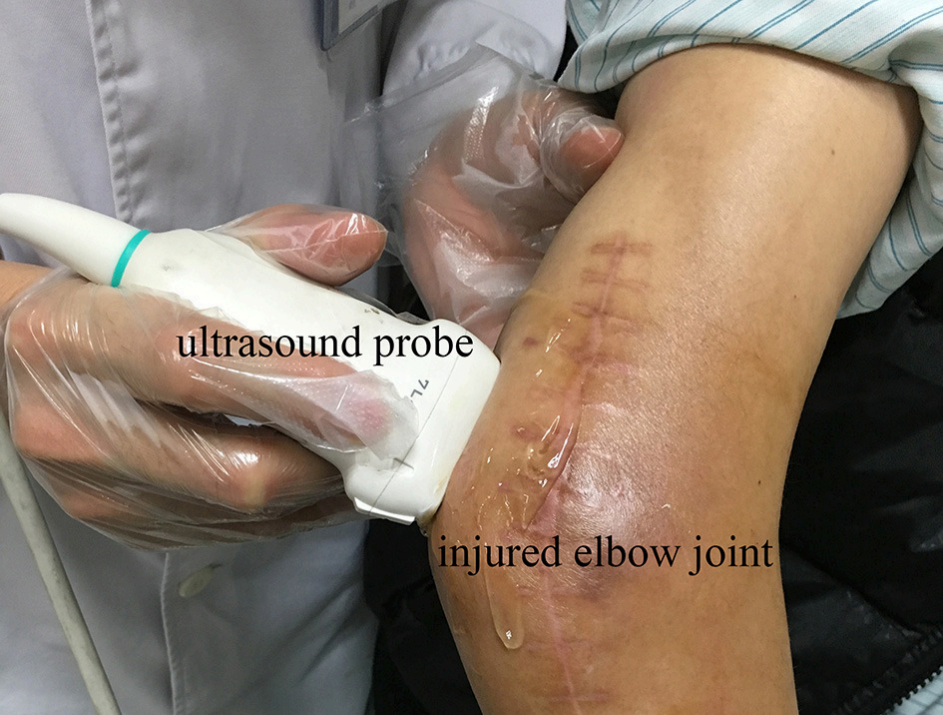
Longitudinal ultrasound scanning of HO at the posteriorlateral site of the injured elbow joint of participant No. 1.
